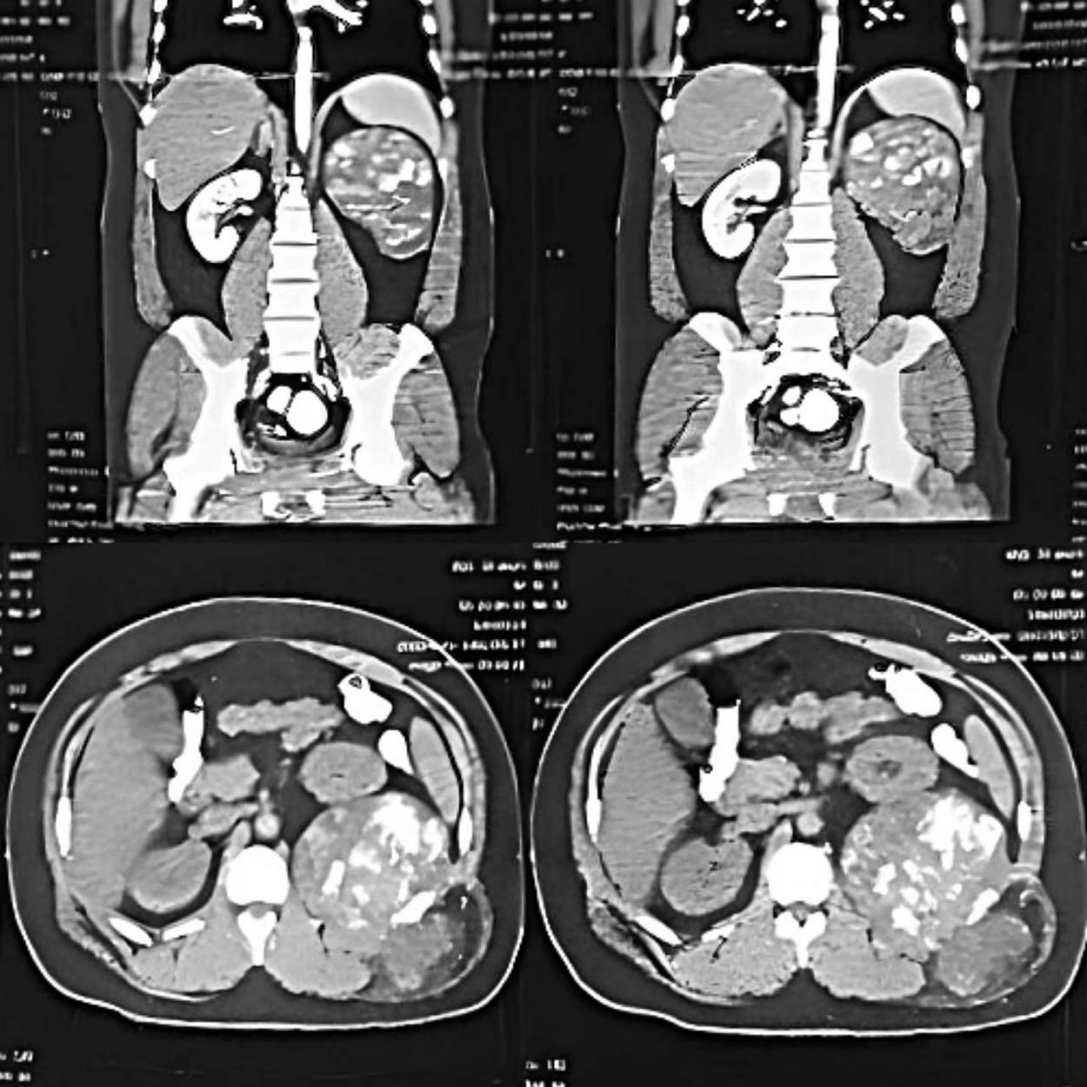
Preoperative computed tomography (CT) scan showed the presence of a lytic expansile lesion with multiple internal high densities from the left lower ribs.
